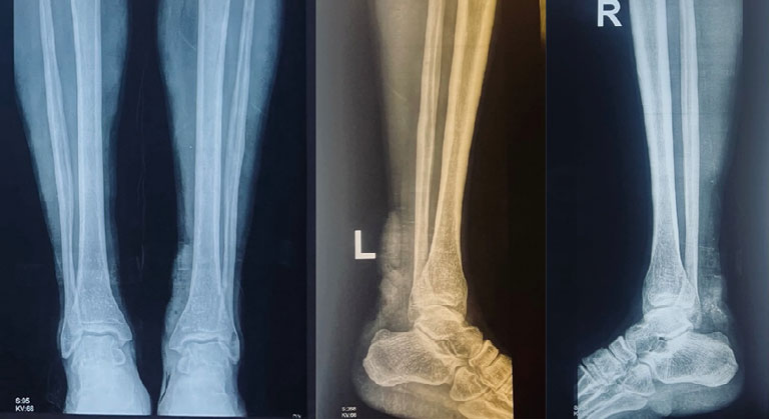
Radiographs of the bilateral ankle anteroposterior and lateral views with disrupted Kager's triangle on the lateral views and with no fractures.
